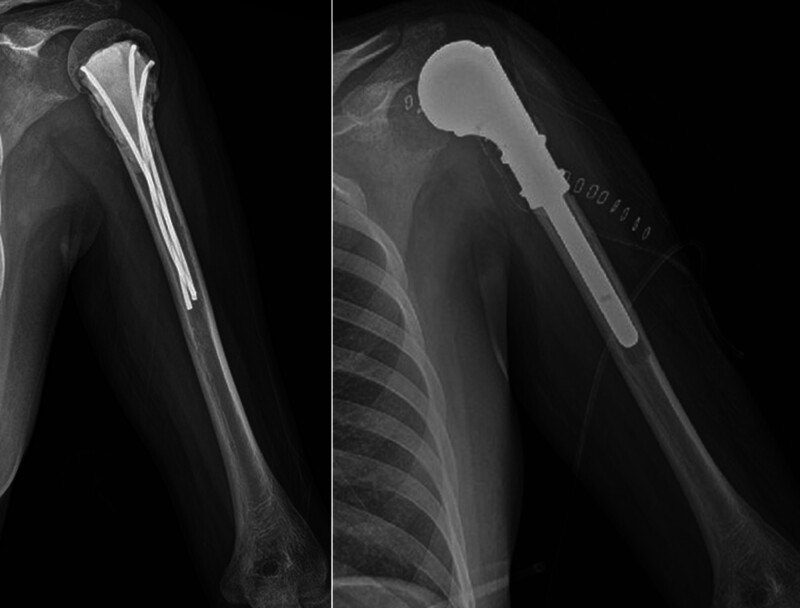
Postoperative images of the left shoulder. Plain radiograph (left) after extended curettage with bone cementing. Plain radiograph (right) after tumor-wide excision and bipolar hemiarthroplasty.
